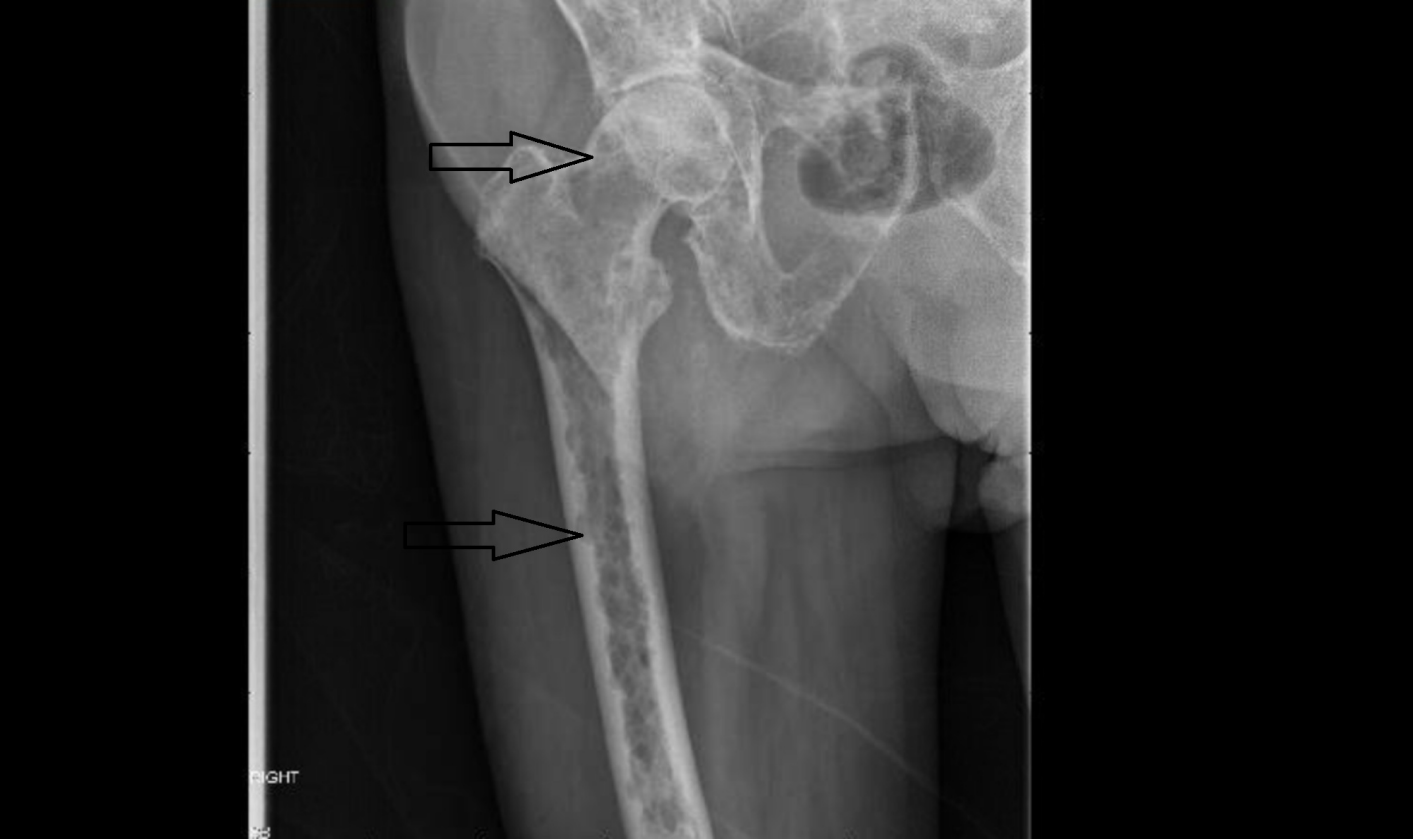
X-ray of the femur Showing multiple lytic lesions marked by arrows.
